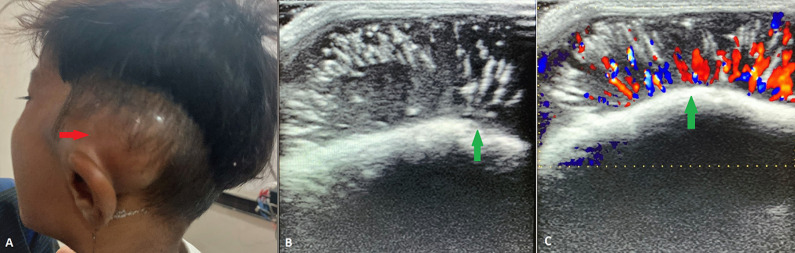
A) clinical image of swelling in post-auricular region above the temporal bone (red arrow); B) ultrasound image showing a hypoechoic mass lesion with sunray appearance (green arrow); C) color Doppler image showing increased vascularity in a linear branching pattern of sunray appearance (green arrow)
